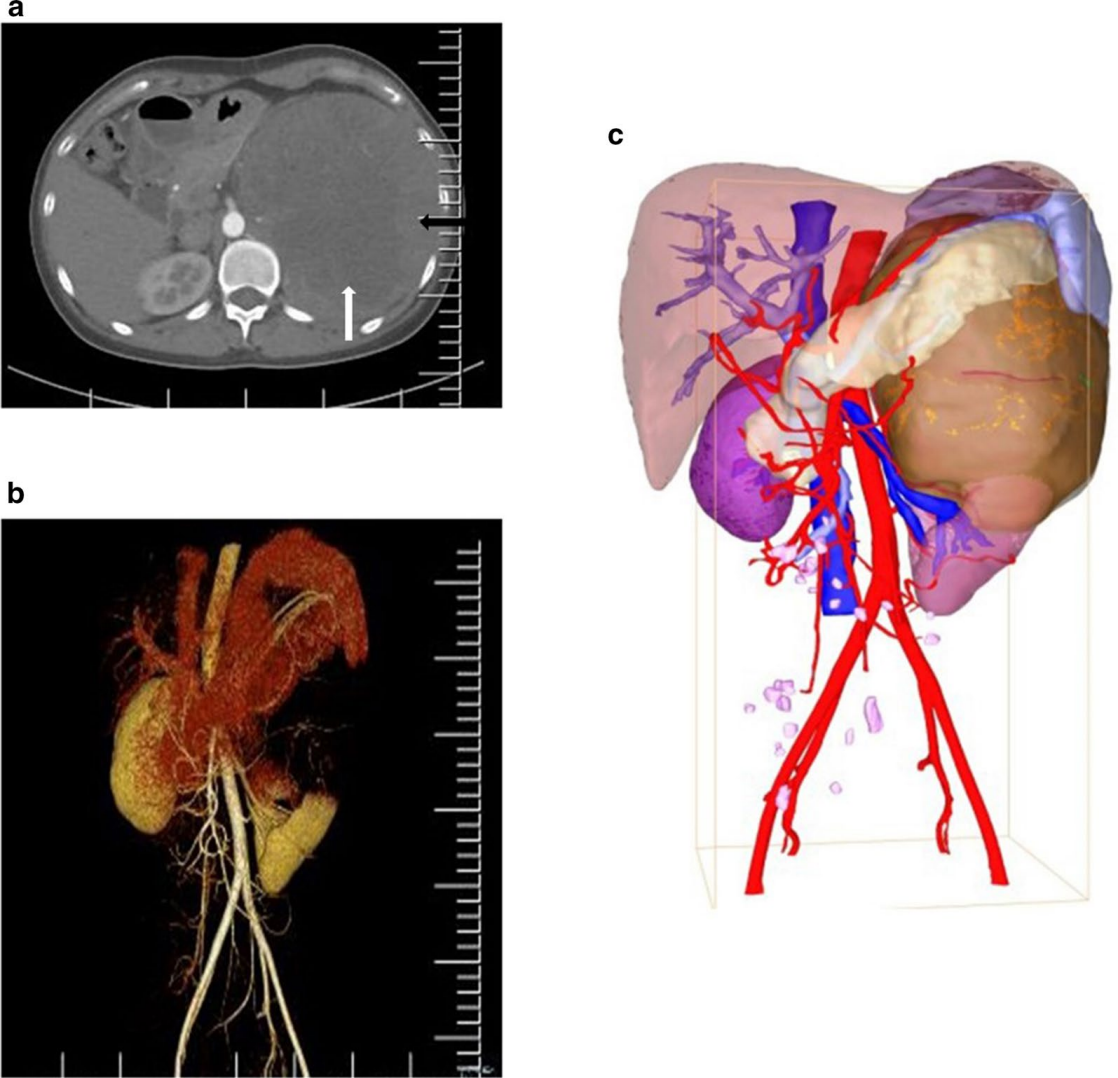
CT scan and three-dimensional imaging. Enhanced computed tomography (CT) revealed a hypoechoic mass about 18 cm × 14.5 cm × 14 cm arising from the left renal. a Cross-section. The black arrows represent the enhanced solid tumors and the white arrows represent non-enhanced tumor necrosis components. b Angiography of bilateral renal. c Three-dimensional imaging of the neoplasm
